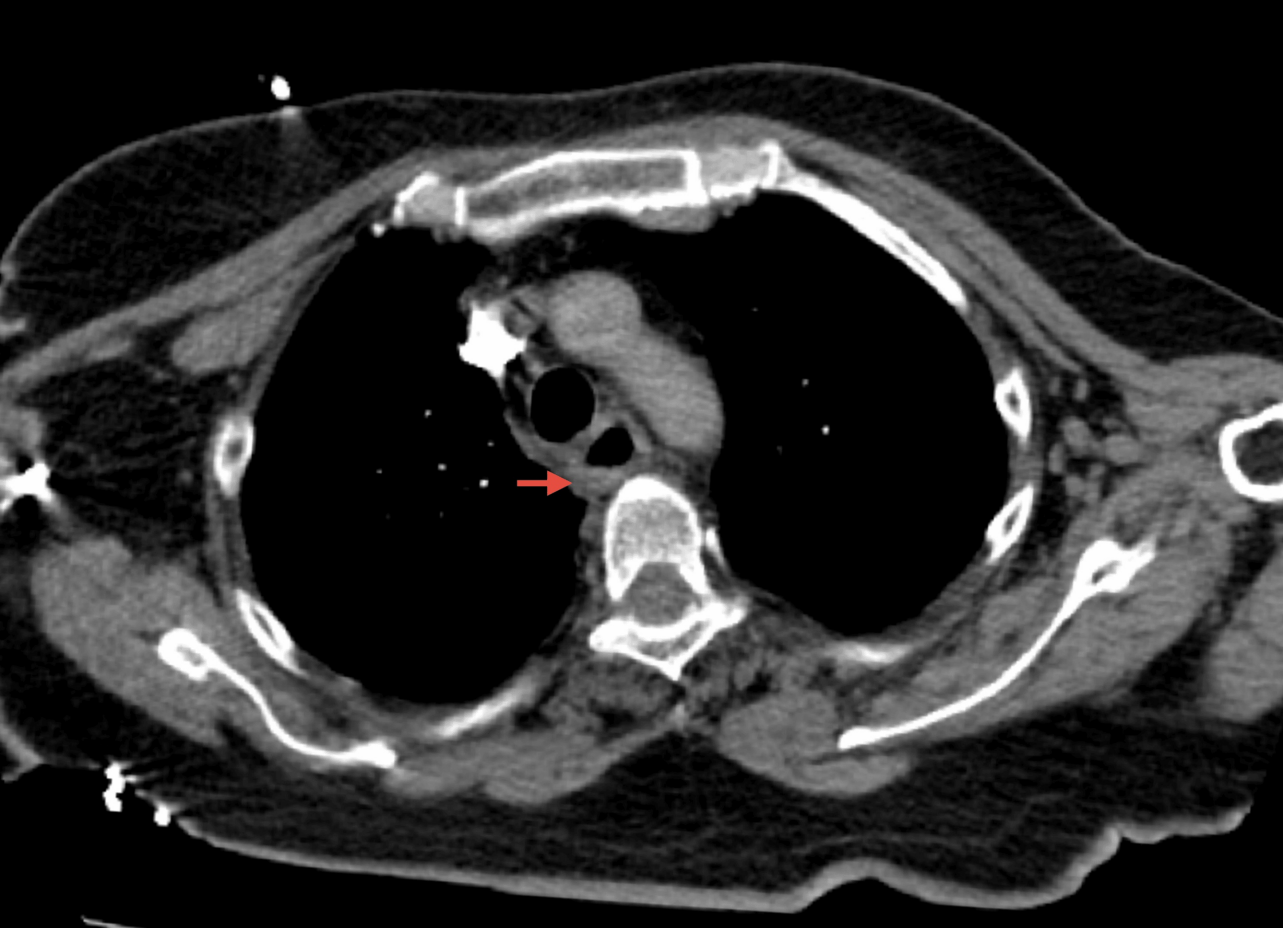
Chest CT scan (axial) revealing thickening of the esophagus (red arrow) without signs of perforation
